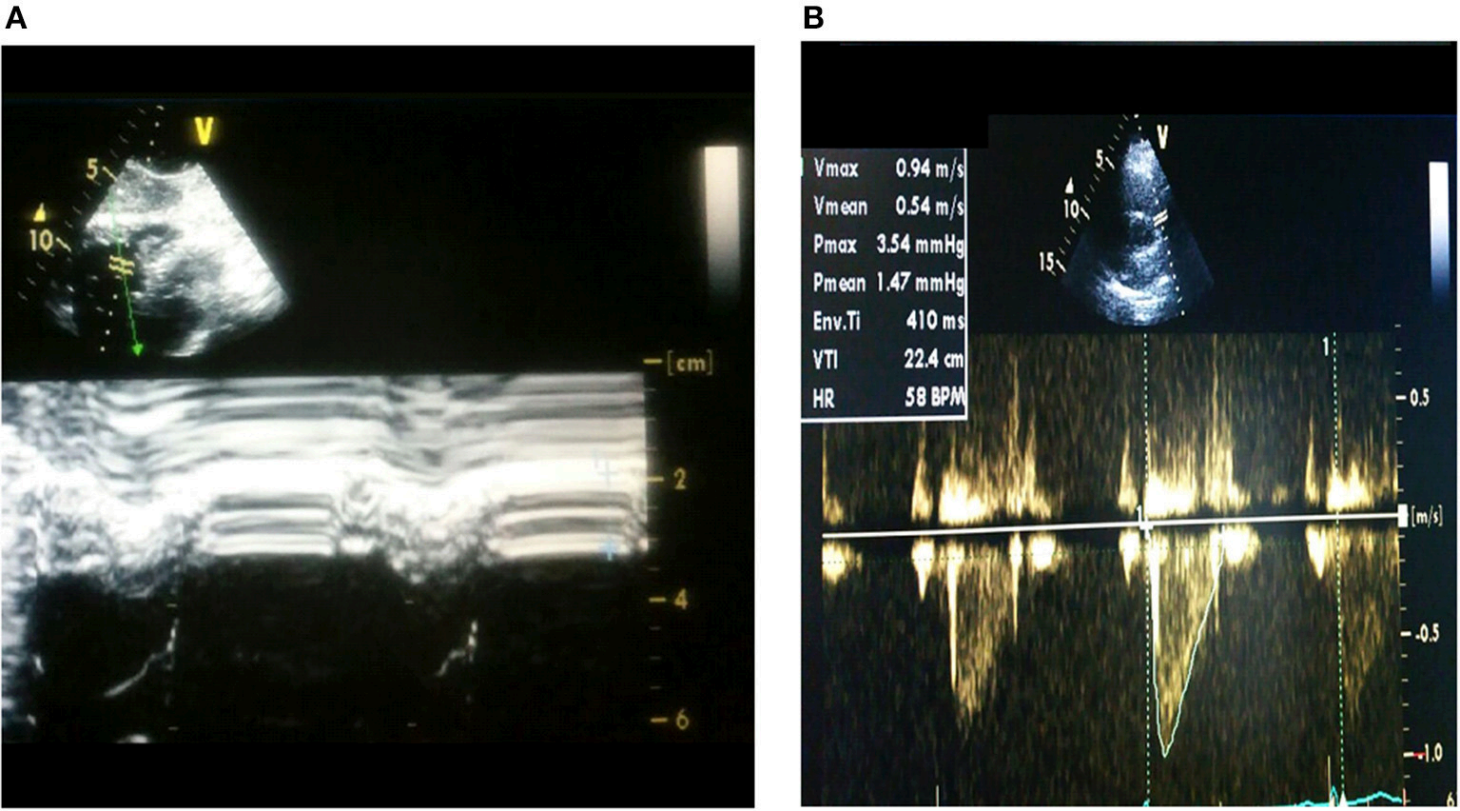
Representative echocardiographic images (A) right ventricular hypertrophy and (B) Acceleration curve of pulmonary flow at pulmonary artery outflow tract.
